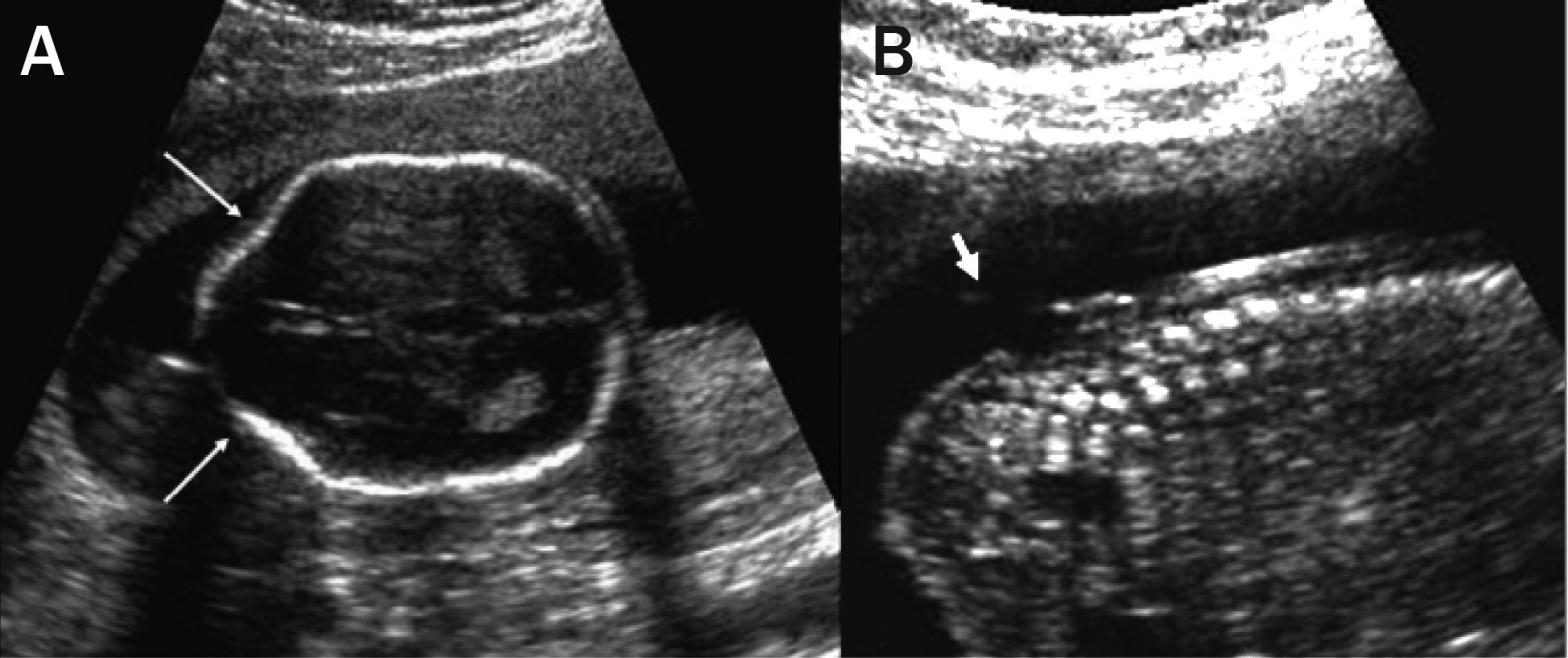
Lemon sign. Axial sonographic image of the fetal head at the level of the biparietal diameter (A) shows inward concavity of both frontal bones (arrows) instead of the normally seen convexity. This gives the head the appearance of a lemon. Sagittal sonographic image of the fetus (B) shows a meningomyelocele (thick arrow) in the lumbar region. Reproduced with kind permission of the Indian Journal of Radiology and Imaging (33).
